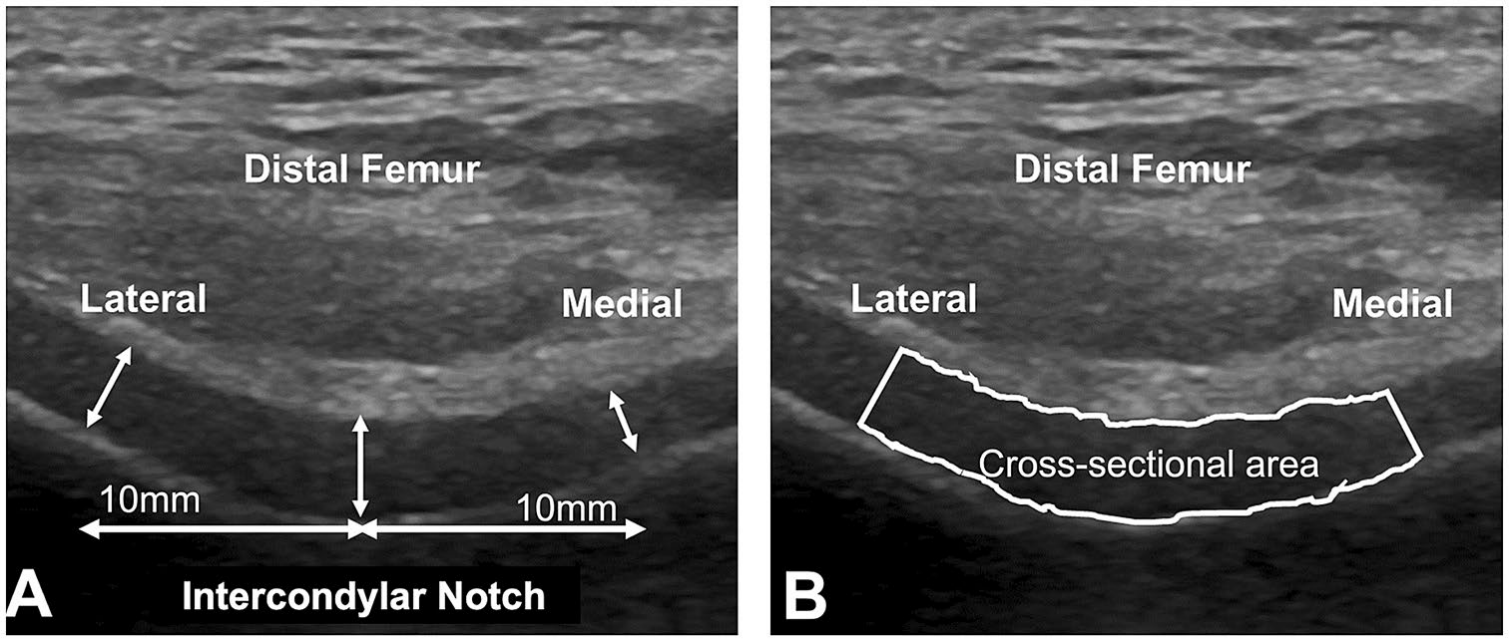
Ultrasonographic image of the anterior femoral articular cartilage: (A) Articular cartilage thickness. (B) Articular cartilage partial area.
